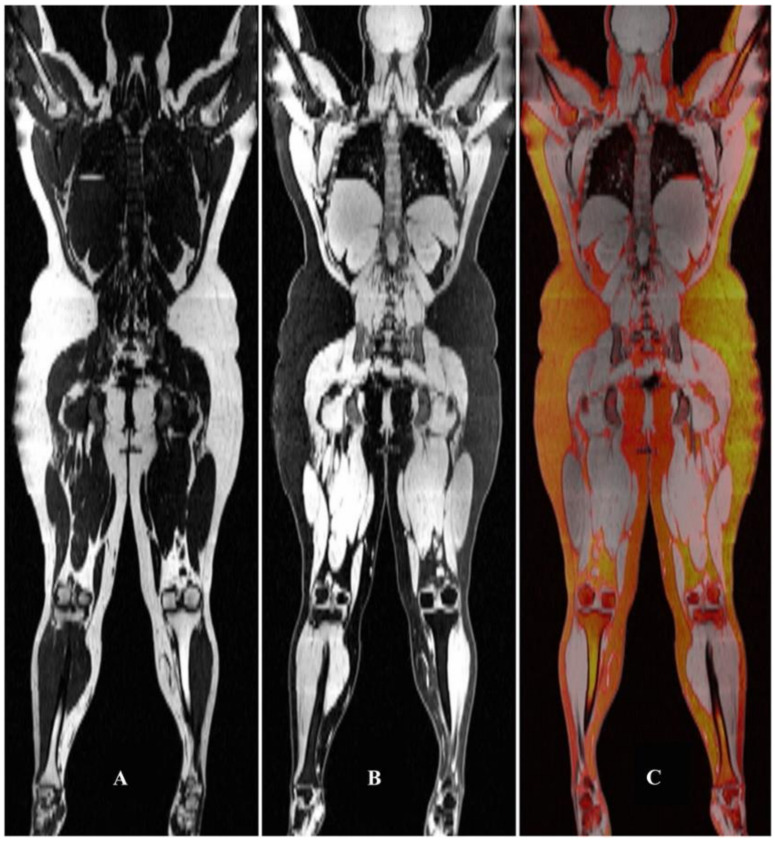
Separated fat (A) and water (B) MRI of an obese individual. Panel (C) depicts the fat overlayed in color on the water grayscale image. From Seabolt et al. (2015), with permission.
